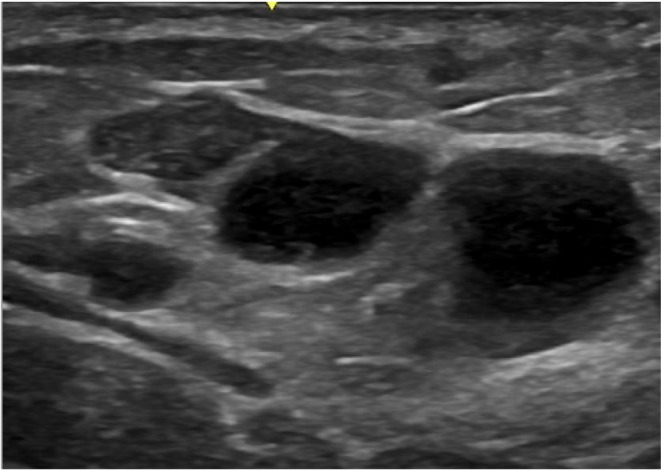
First polyacrylamide gel. Ultrasound image of polyacrylamide gel (PAAG) in the gluteal region, showing conglomerated hypoechoic pseudocystic areas with internal hyperechoic deposits, occasionally adjacent to anechoic vesicles.
